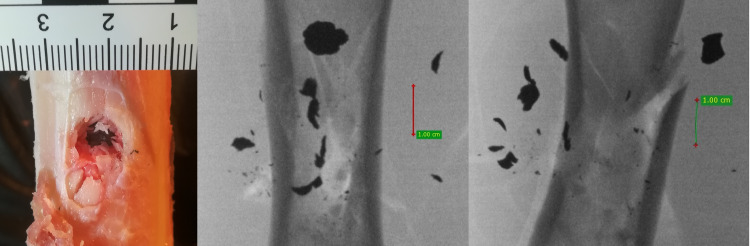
H&N Silverpoint airgun pellet cal. 6.35 mm, on the left: A-P X-ray, in the centre: image of entry damage, on the right: lateral X-ray. Shot to the lateral surface of the femoral shaft.
